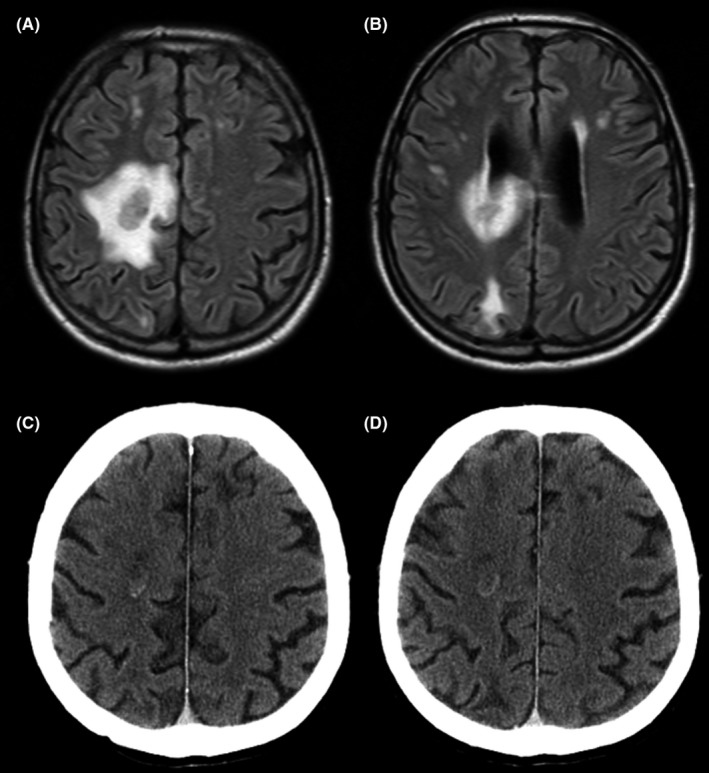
A and B, FLAIR cerebral MRI sequence of October 2007, in favor of a pre‐rolandic right frontal lymphomatous recurrence of 23 × 19 × 15 mm of the supra‐ventricular right white matter with perilesional edema and discrete mass effect on the roof of the right lateral ventricle. C and D, cerebral computed tomography of January 2008, displaying an annular enhancement measuring approximately 19 mm (antero posterior axis) by 17 mm (height) of the right semioval center. A discrete perilesional hypodensity is associated, no mass effect on the adjacent structures is observed, notably there is no abnormality of the ventricular system, which is symmetrical. No evolution is detected after comparison with the MRI of October 2007. The patient did not receive an MRI for cerebral evaluation in 2008 because of the development of claustrophobia contraindicating this procedure
